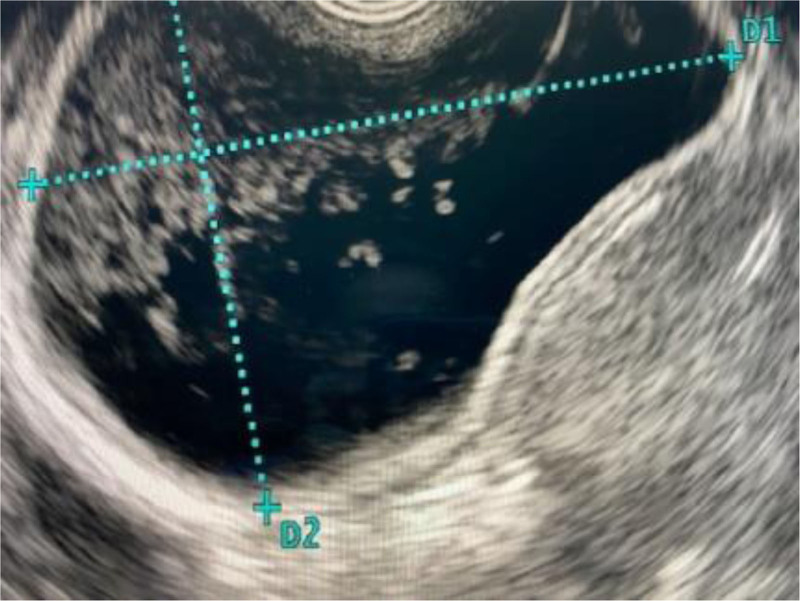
Fifteen-month-old with abdominal pain and vomiting, US and CT showed a mass in the duodenum. EGD showed compression of duodenum, and EUS showed duplication cyst with debris as shown in this image. EUS = endoscopic ultrasound.
